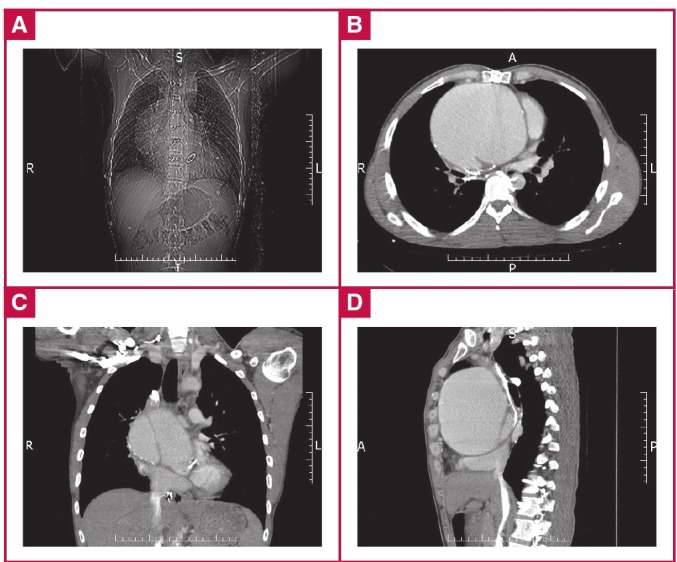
Mediastinal widening seen in pre-operative chest radiography (A). Pre-operative thoracic CT scan: axial (B), coronal (C) and sagittal (D) views showing the giant dissecting aortic aneurysm of 13.25 cm in diameter.
